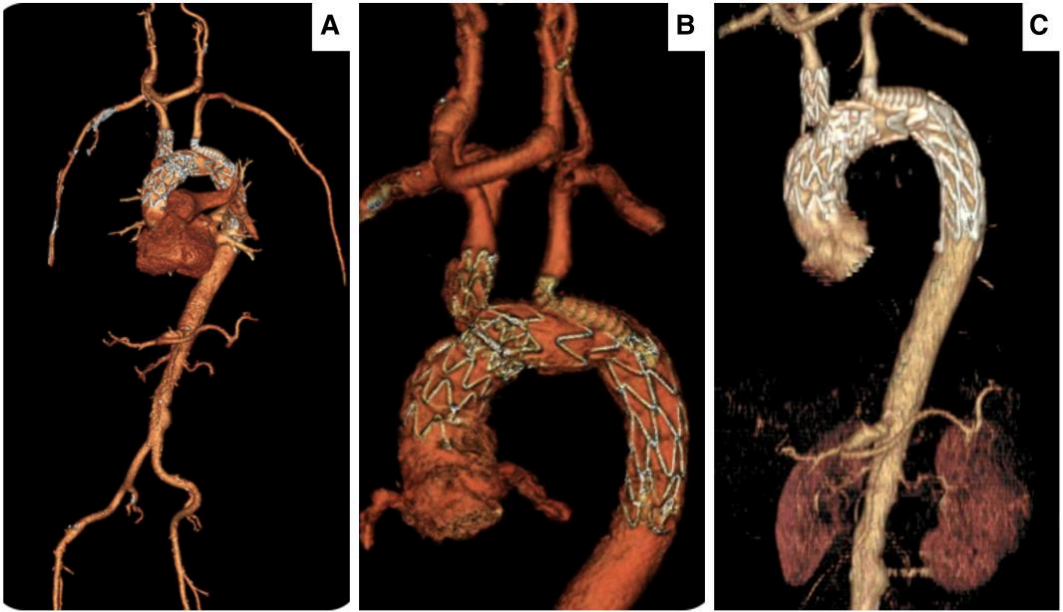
Post-operative 3D reconstructions of the computed tomography angiography scans, with no evidence of graft migration, twist, or endoleaks. (*A*) At 6-week follow-up, all the great vessels of the head and neck were patent. (*B*) At 6-month follow-up, the size of the aneurysm was 6.3 cm. (*C*) At 1-year follow-up, the size of the aneurysm was 5.8 cm.
